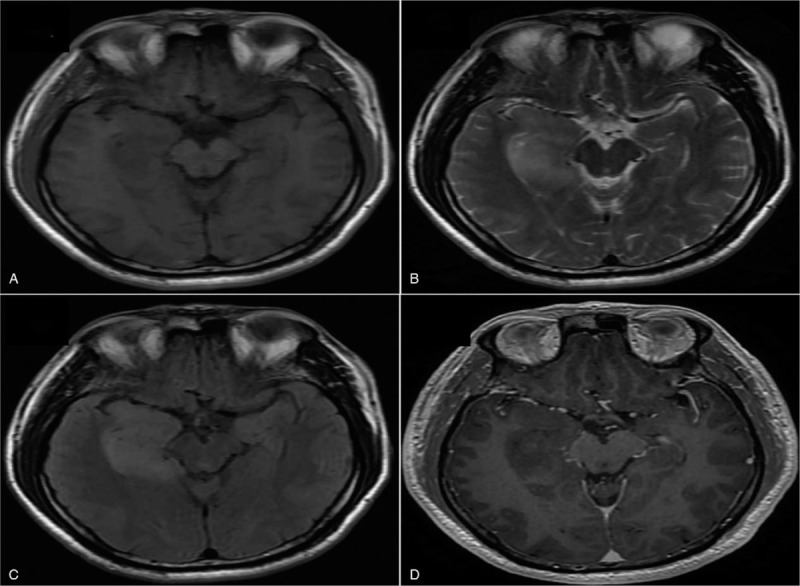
Magnetic resonance images of the case. A diffuse lesion located in hippocampal formation of right anteromedial temporal lobe without mass formed, with slight surrounding swelling, and disappearance of the sulci. The lesion displayed (A) hypointensity on T1-weighted images, hyperintensity on (B) T2-weighted images and (C) T2 fluid attenuated inversion recovery, and (D) nonenhancement.
